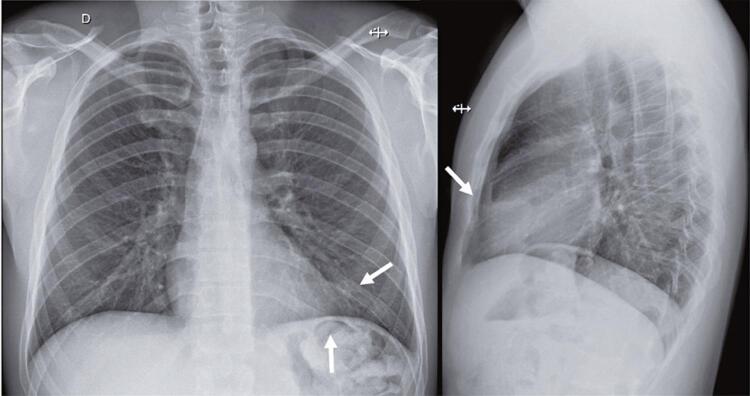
Chest radiograph: elevation of the left dome of the diaphragm and blurring of the cardiac silhouette in the posteroanterior view and opacity of the anterior base of the thorax in the lateral view (white arrows)
